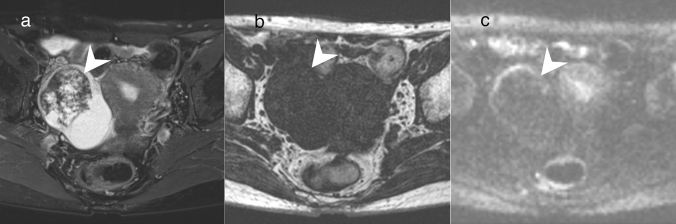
Cystic tumor with solid components showing hypointensity on T2WI and DWI. a–c Fibroma in a woman in her 40 s. A solid and cystic mass is present in the left ovary. The solid components are hypointense on FS-T2WI, comparable to skeletal muscle (arrowhead) (a). The solid components are hypointense on FS-T1WI, comparable to skeletal muscle (arrowhead) (b). The solid components are hypointense on high b value DWI (arrowhead) (c)
